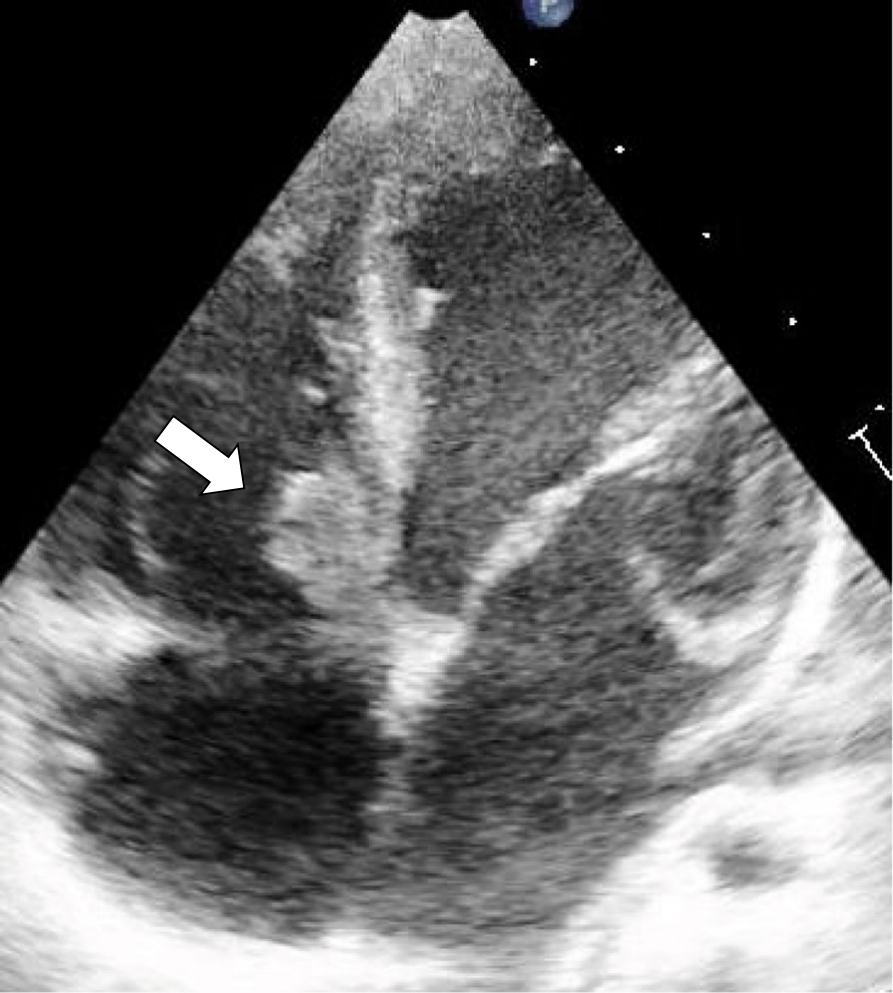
Apical four-chamber view. Vegetation on membranous septal aneurysm (arrow)
